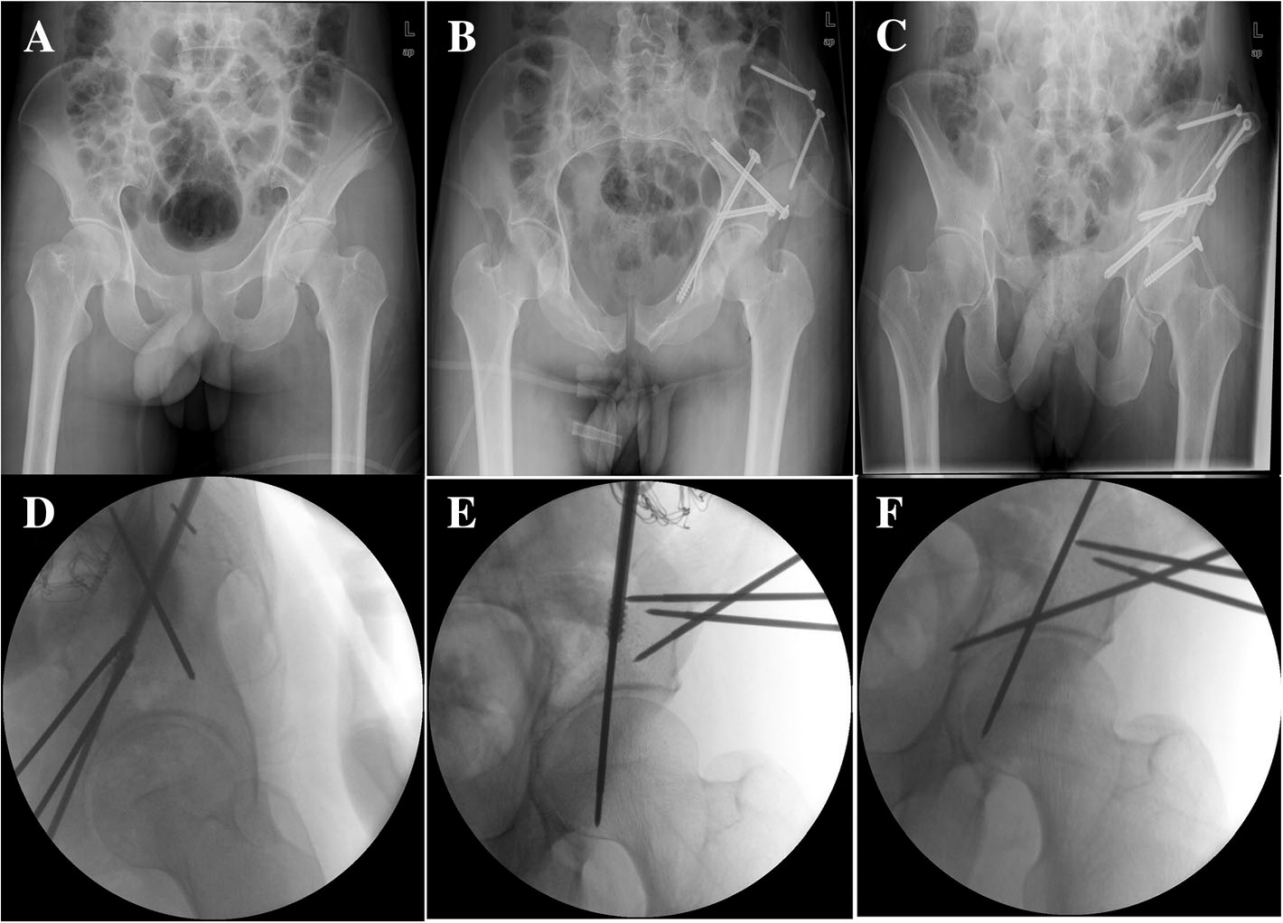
The standard procedure based on this technique. a Preoperative anteroposterior view of pelvic; b Postoperative inlet pelvic view; c Postoperative outlet pelvic view; d Intraoperative lateral views for placing K-ware; e and f intraoperative iliac oblique radiography view for placing K-ware
